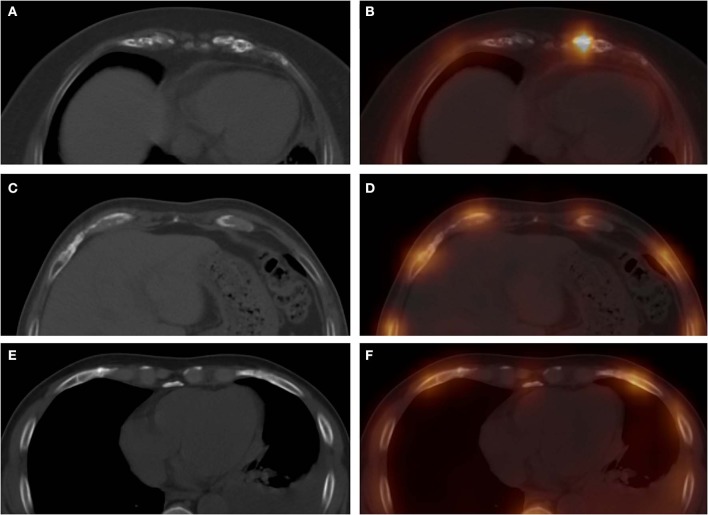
These images were obtained from three patients in the CG. The images (A, CT; B, fusion) were obtained from a 57-year-old woman with breast cancer, which revealed a high-uptake focus in the left seventh costal cartilage with mild irregular calcification. Computed tomography, SPECT, and SPECT/CT all showed false-positive diagnoses in this patient. The images (C, CT; D, fusion) were obtained from a 55-year-old man with nasopharyngeal carcinoma, which revealed a moderate-uptake focus in the right eighth costal cartilage with normal calcification. Single-photon emission computed tomography showed a false-positive diagnosis in this patient. The images (E, CT; F, fusion) were obtained from a 62-year-old man with left lung cancer, which revealed mild irregular calcification in the right sixth costal cartilage, with normal tracer distribution. Computed tomography showed false-positive diagnosis in this patient.
